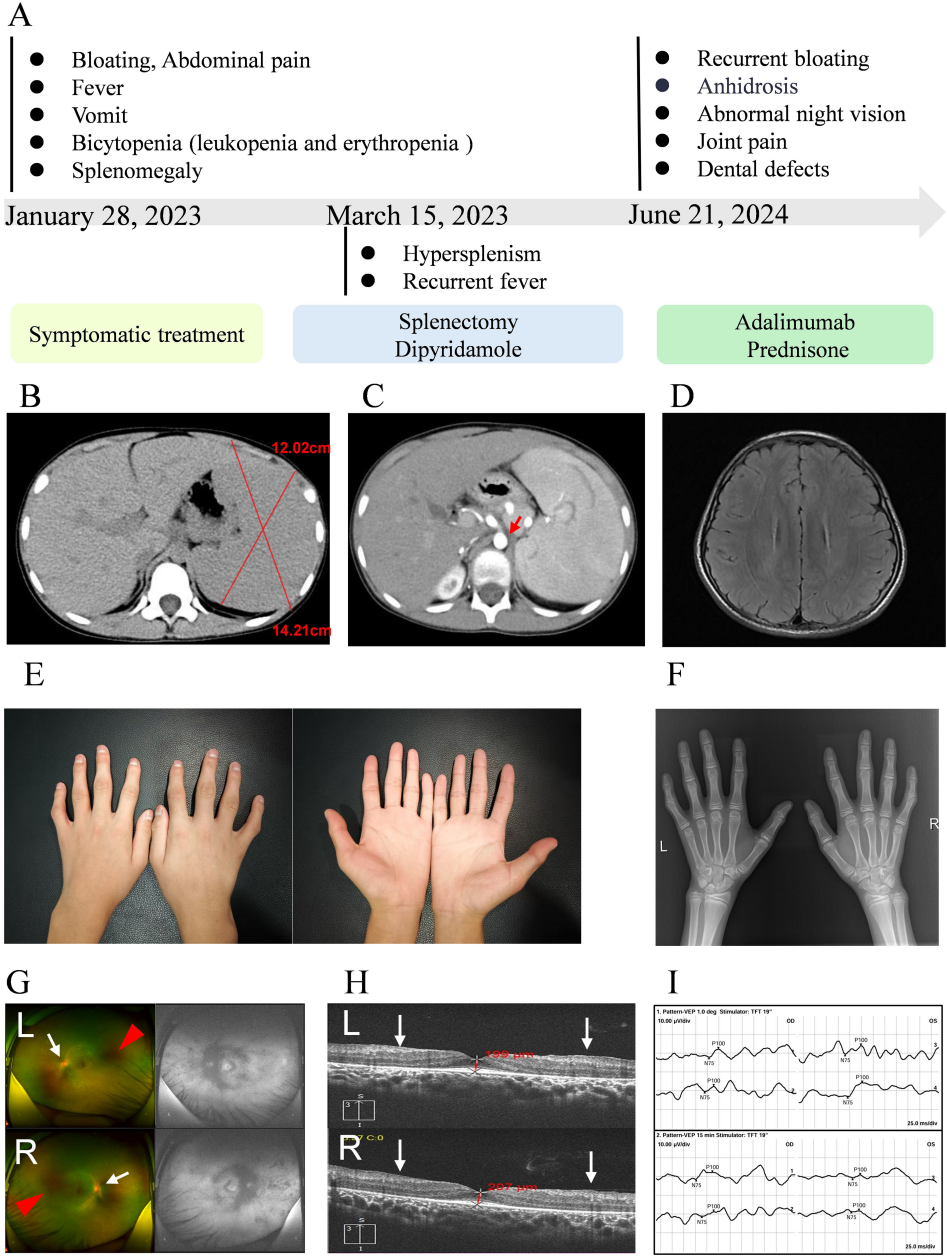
Clinical progression and phenotypic features. (A) Disease trajectory and temporal evolution. (B, C) Cross-sectional abdominal imaging; red arrows indicate dilation of the celiac trunk. (D) Cranial MRI. (E) Photographs of both hands. (F) Radiograph of the hands. (G) Fundus photographs (upper: left eye, lower: right eye); white arrows indicate blurred optic disc margins, red arrows indicate extensive hypofluorescent areas around the optic disc and nasal periphery. (H) Optical coherence tomography (OCT; upper: left eye; lower: right eye), white arrows indicate structural retinal abnormalities. (I) Visual evoked potential (VEP) testing (upper: 1.0-degree pattern VEP; lower: 15-minute pattern VEP).
